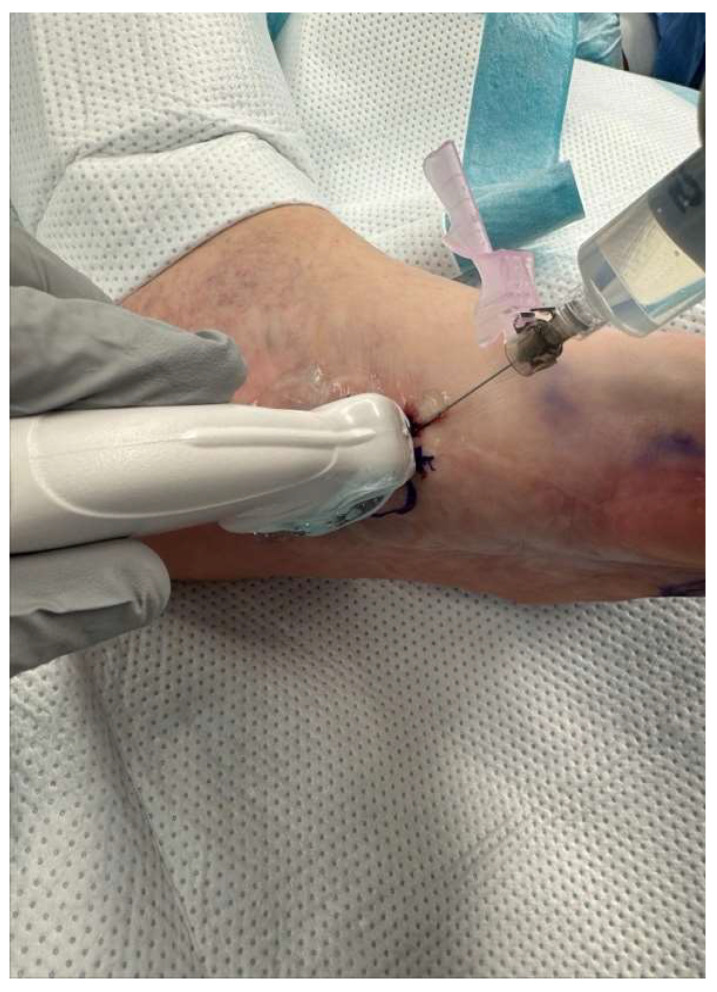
Ultrasound-guided injection of hyaluronidase, triamcinolone acetonide, and lidocaine 1% plain into fibroma.
